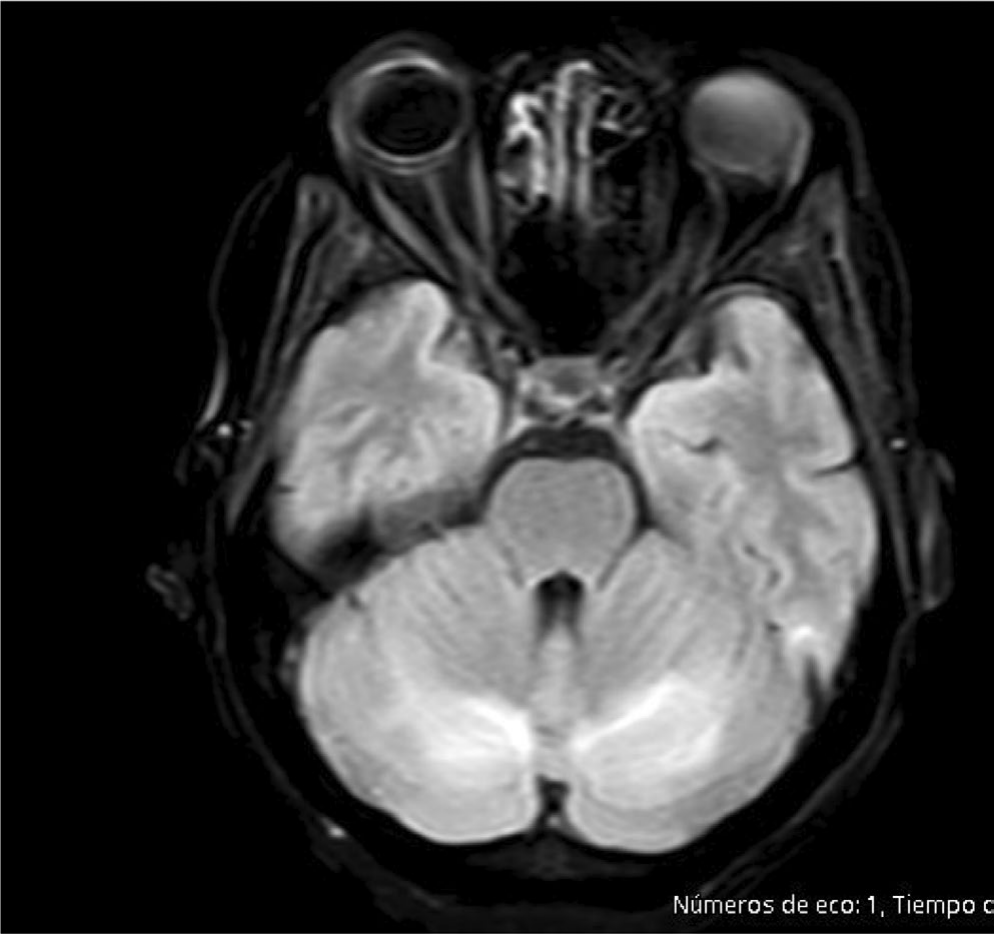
FIGURE 2 Hypersignal in both cerebellar hemispheres, FLAIR.
